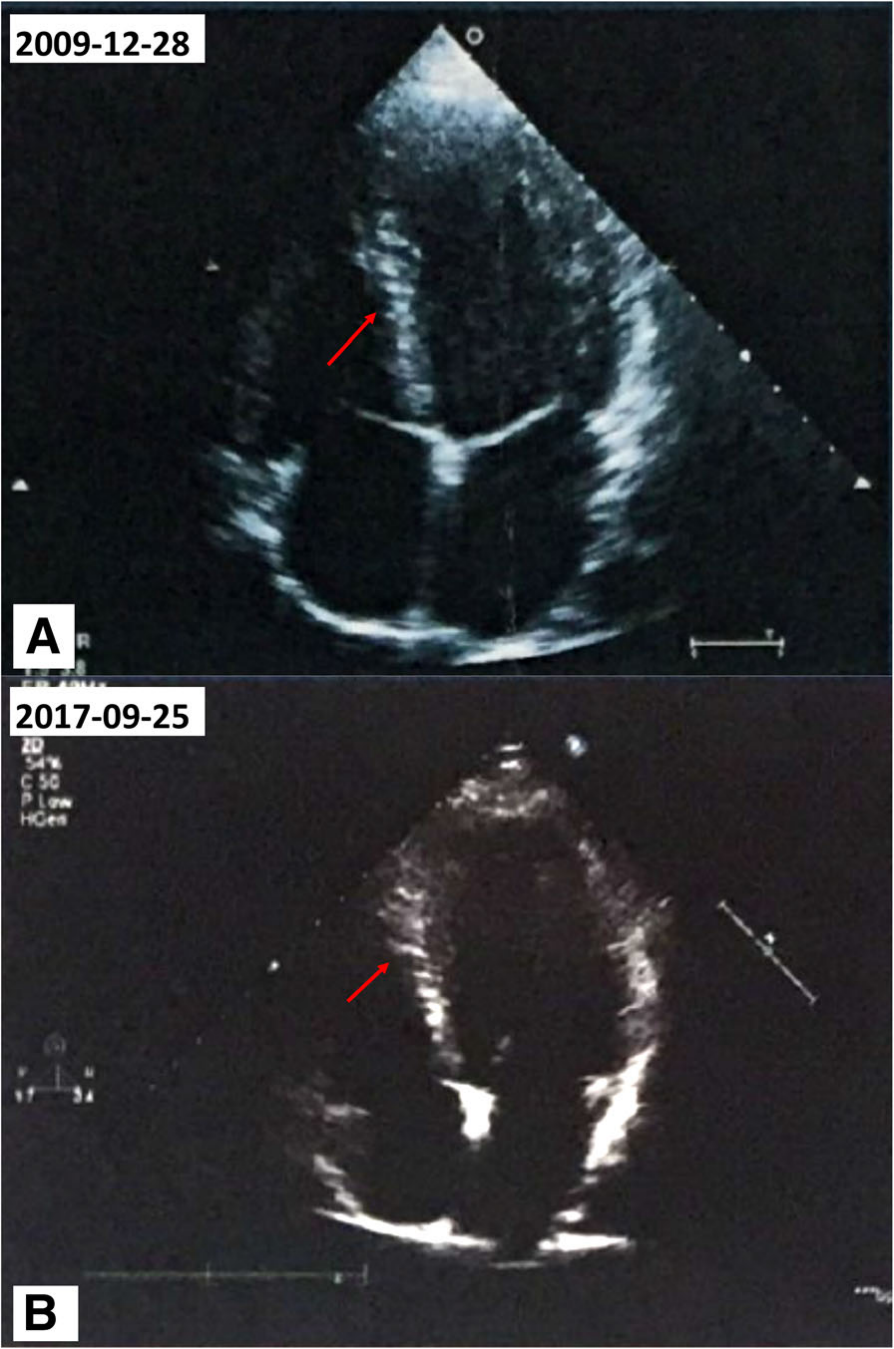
Echocardiography showed increasingly thickened interventricular septum from 10 mm (a) to 13 mm (b) (*red arrowheads*), enlarged left atrium and ventricle, and reduced left ventricular diastolic function
